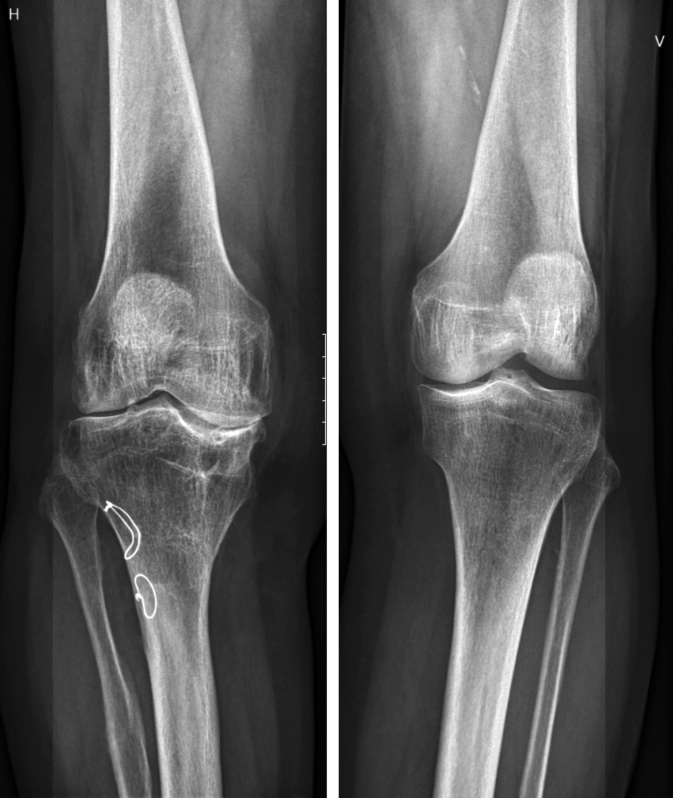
Radiographs at 65 years follow-up, showing moderate osteoarthritis of the right knee.
